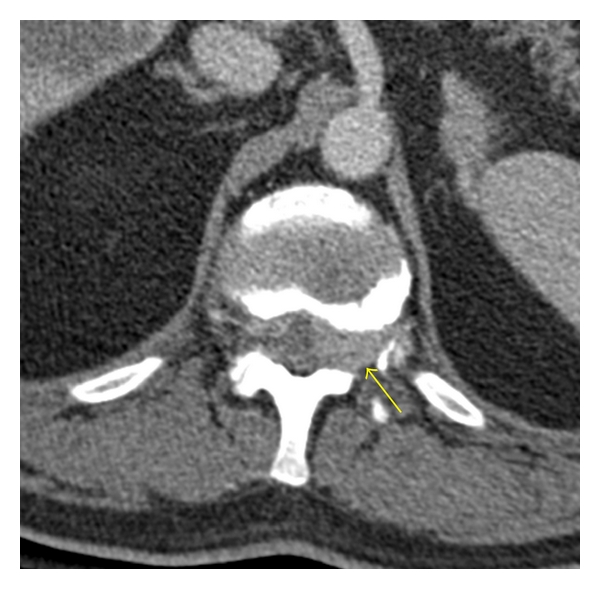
Axial CT in soft tissue algorithm displays slightly hyperdense soft tissue in the ventrolateral epidural space filling the left neural foramen (yellow arrow) and causing mass effect on the thecal sac.
